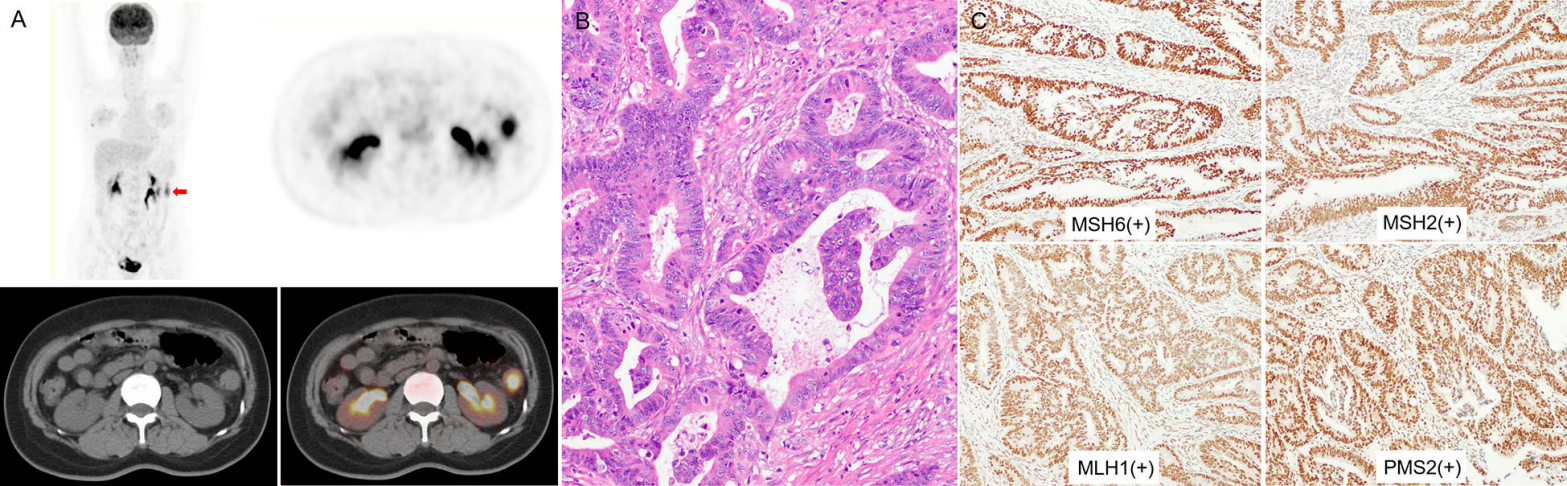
(A) a 48-year-old woman with left colon cancer. ^18^F-FDG PET/CT showed hypermetabolism in the descending colon (arrow; SUV_max_ 9.86; HI_60%_ 1.38). (B) The pathological diagnosis was moderately differentiated CRC, without mucinous component. (C) IHC analysis results for MMR proteins were MSH6(+), MSH2(+), MLH1(+), and PMS2(+), indicating MSS.
